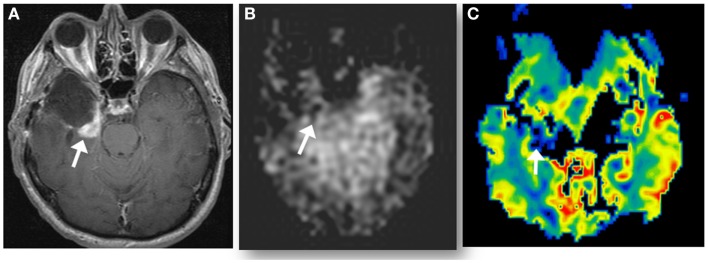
A new enhancing lesion appeared along medial margin of right temporal resection cavity 2 months following completion of chemoradiation (A), without evidence of neither elevated CBF on ASL perfusion (B), nor elevated rCBV (C) on DSC-MRI. The lesion was confirmed as pseudoprogression on subsequent imaging.
